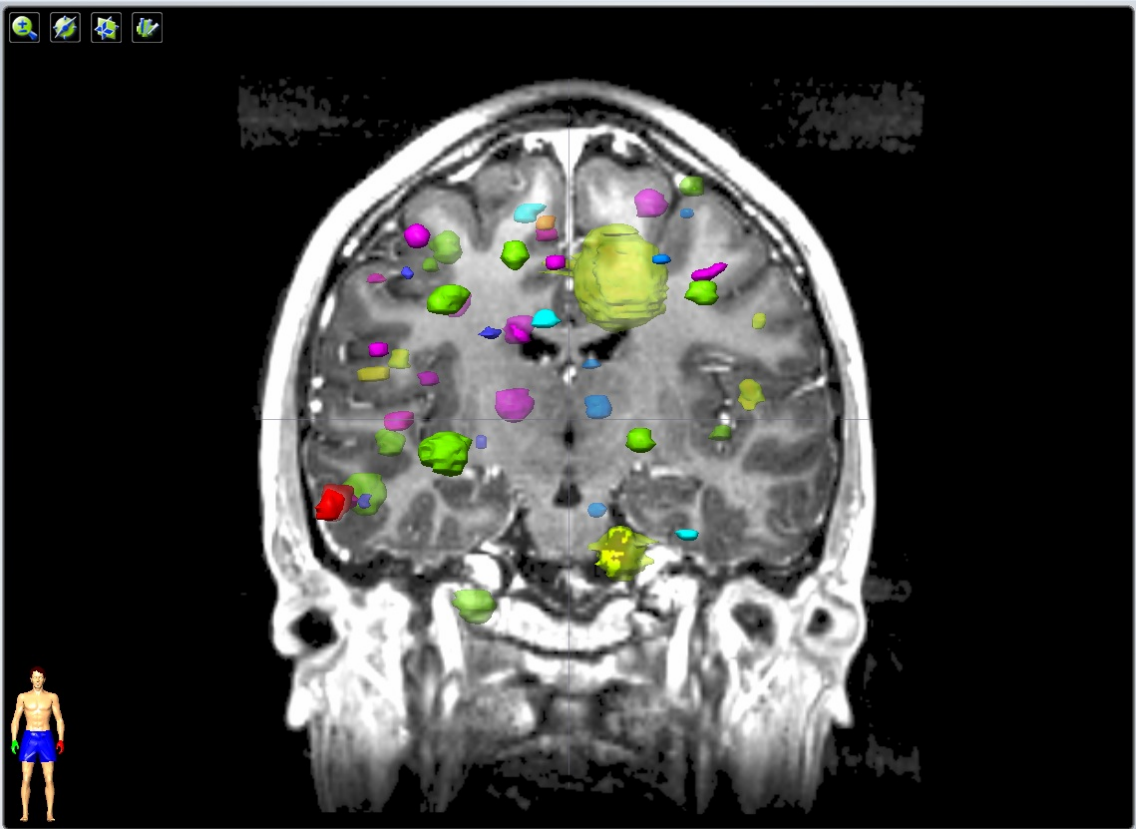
The treated lesions superimposed on pre-SRS MRI scan Forty-seven lesions superimposed on a coronal MRI image plane scanned prior to the first course of SRS. The lesions are color-coded; each color represents the lesions treated in the same course of stereotactic radiosurgery.
